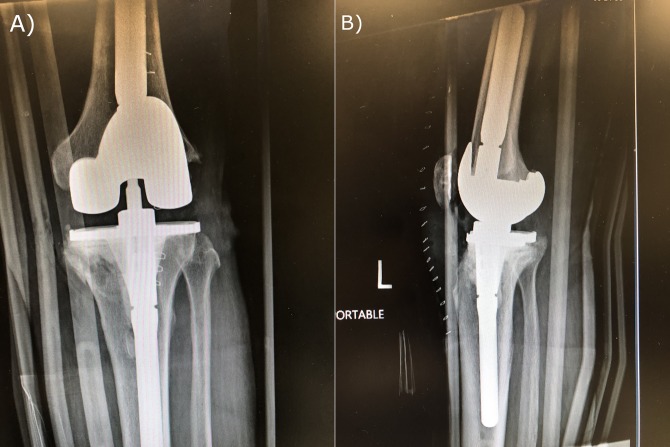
Radiograph showing postoperative AP (A) and lateral (B) views of the left knee after a total knee arthroplasty revision taken 1 day after surgery (November 2, 2018).
